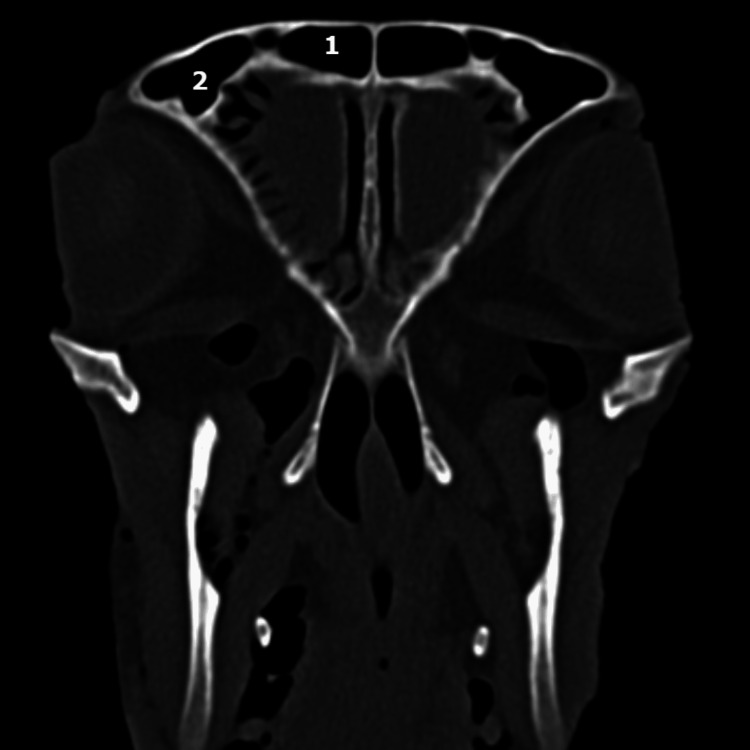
Coronal CT scan: frontal sinus 1: medial chambers of frontal sinus; 2: lateral chambers of frontal sinus.
